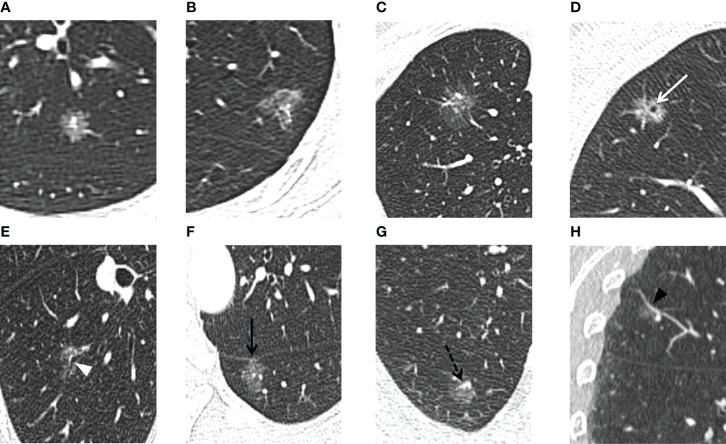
For the PSNs included in our study, thin-section CT radiographic features were evaluated. The lesion shape is evaluated as (A) round/oval or (B) irregular. The lesion border is evaluated as (A, B) defined and (C) undefined. (D) Vacuole sign: MIA in the right upper lobe exhibits a bubble-like lucency within the nodule (white arrow). (E) Air bronchogram sign: MIA in the right lower lobe shows air-filled bronchi present inside the lesion (white arrowhead). (F) Pleural indentation: MIA in the left lower lobe shows pleural indentation adjacent to the oblique fissure (black arrow). (G, H) Microvascular sign: IAC in the right upper lobe shows a small adjacent pulmonary vessel entering the lesion (black dotted arrow). Reverse tracing shows that the blood vessel is a branch of the pulmonary artery. Coronal reconstruction better shows the dilation of the supplying vessel (black arrowhead).
